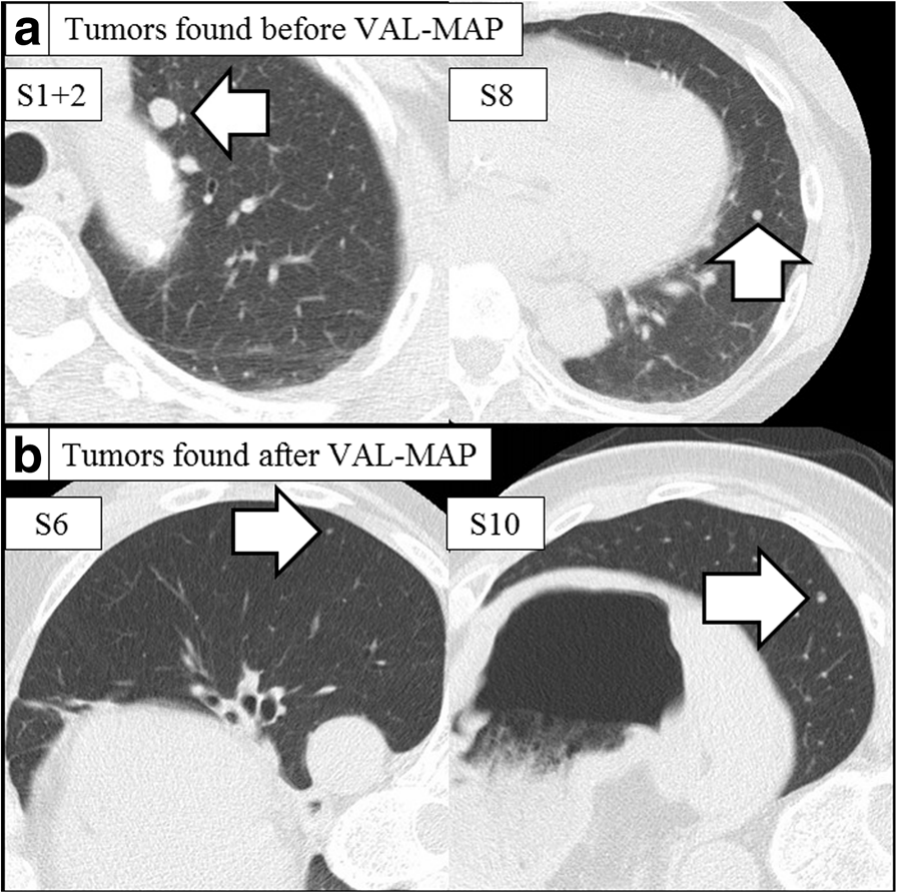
Chest computed tomography images of pulmonary nodules. a Chest computed tomography (CT) images taken before bronchoscopic marking revealing an 8-mm nodule located in S1 + 2 and a 5-mm nodule in S8. b Images taken just after bronchoscopic marking showed two unexpected tumors; a 2-mm nodule located in S6 and a 3-mm nodule in S10. The chest computed tomography was taken in a right decubitus position, facilitating detection of tiny nodules that may have been hidden by the effect of gravity in the pre-VAL-MAP CT scan, which was performed with the patient lying supine
